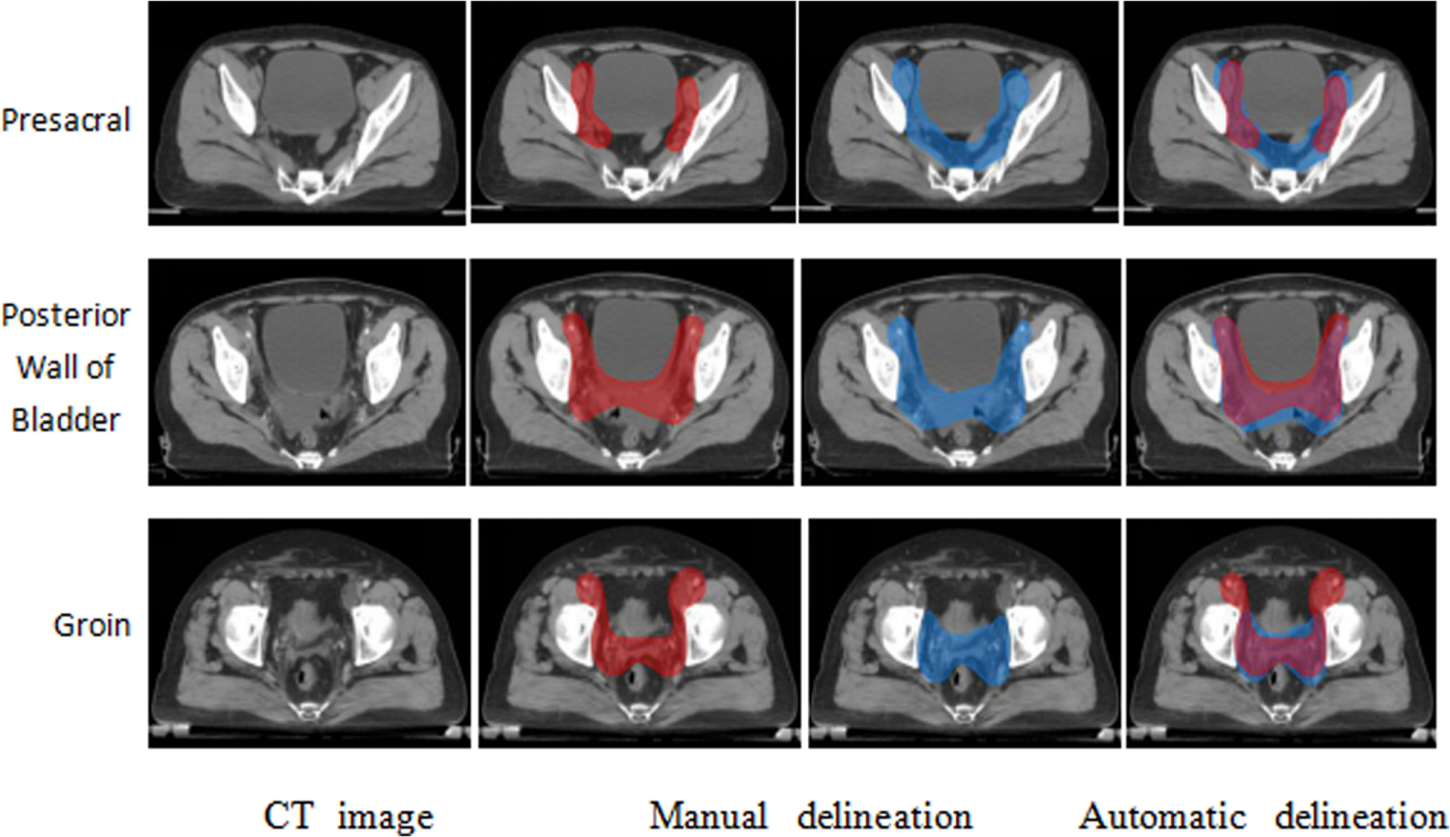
CT cross-sectional images of patients and Comparison of delineations in three conditions of huge difference
